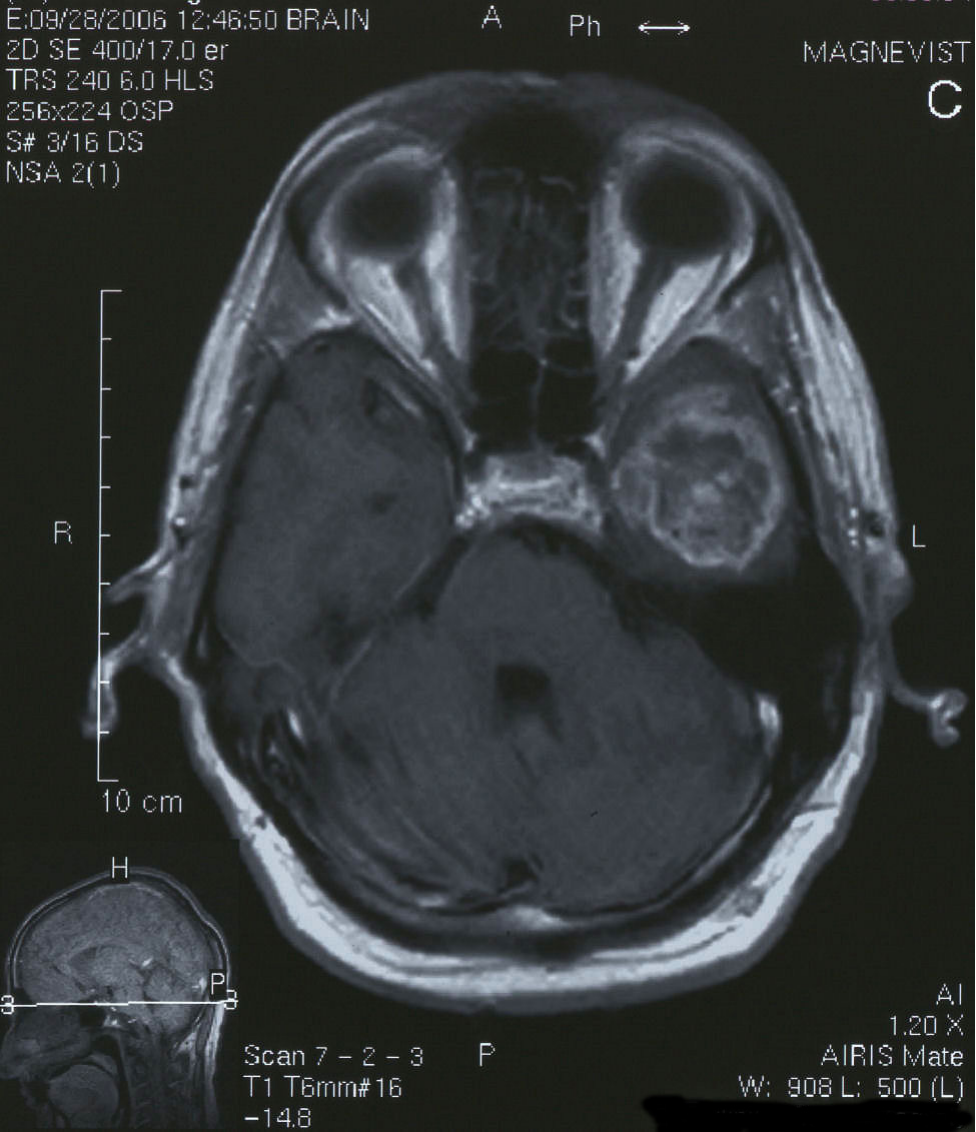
Axial T1 weighted contrast enhanced MRI image demonstrating ring-enhanced lesion of the left temporal lobe
